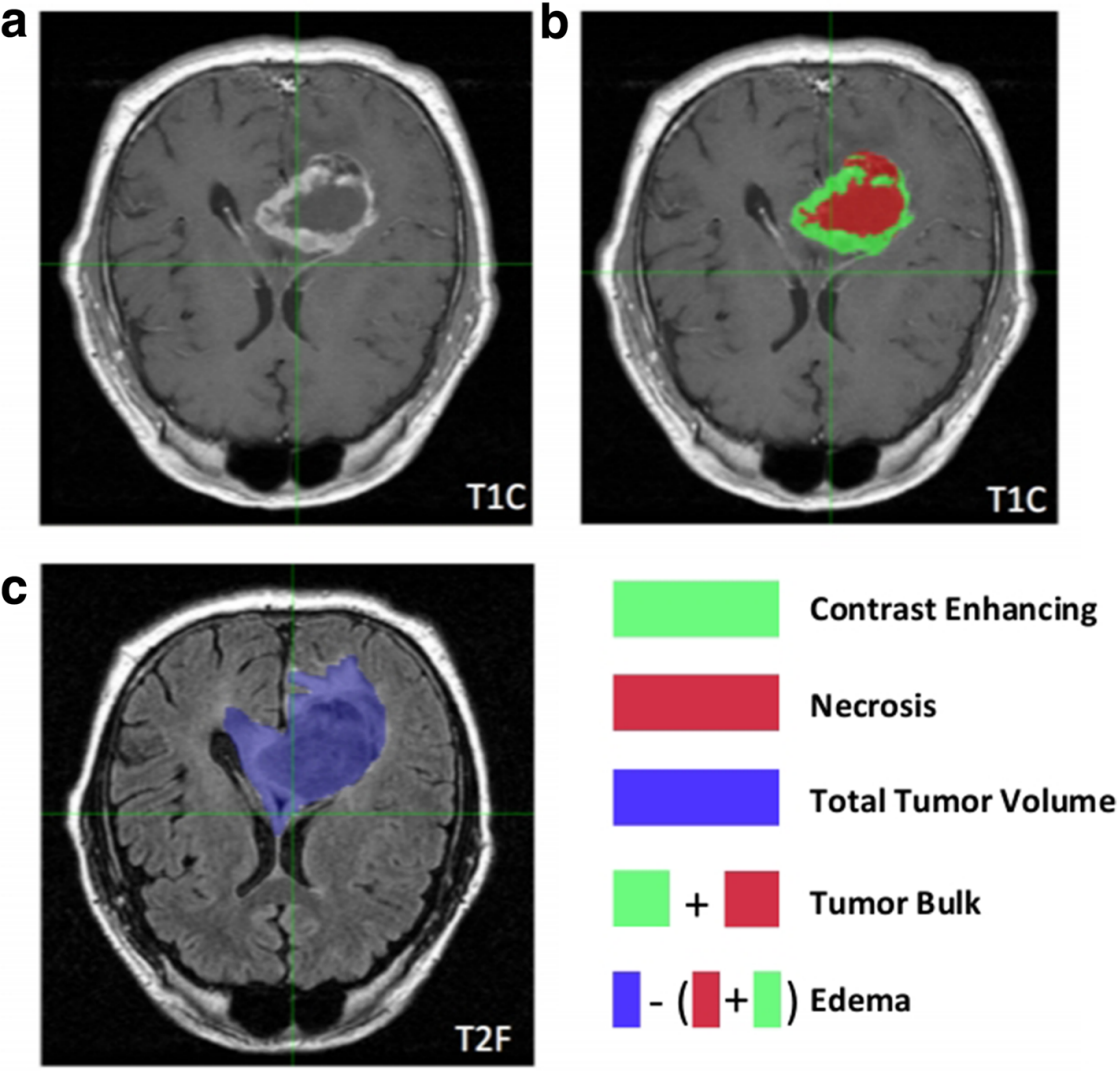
Volumetric phenotype features within the same tumor. Detailed example of a glioblastoma tumor in a patient. (a,b) On T1-weighted post-Gadolinium contrast (T1C) images, a central area of Necrosis is typically surrounded by a Contrast Enhancing ring, both of which can be derived from dark and light regions, respectively. Tumor Bulk represents the addition of these tumor features. (c) The Total Tumor Volume is represented by hyperintensity extracted from T2-FLAIR images. Edema is the difference of Tumor Bulk from Total Tumor Volume
